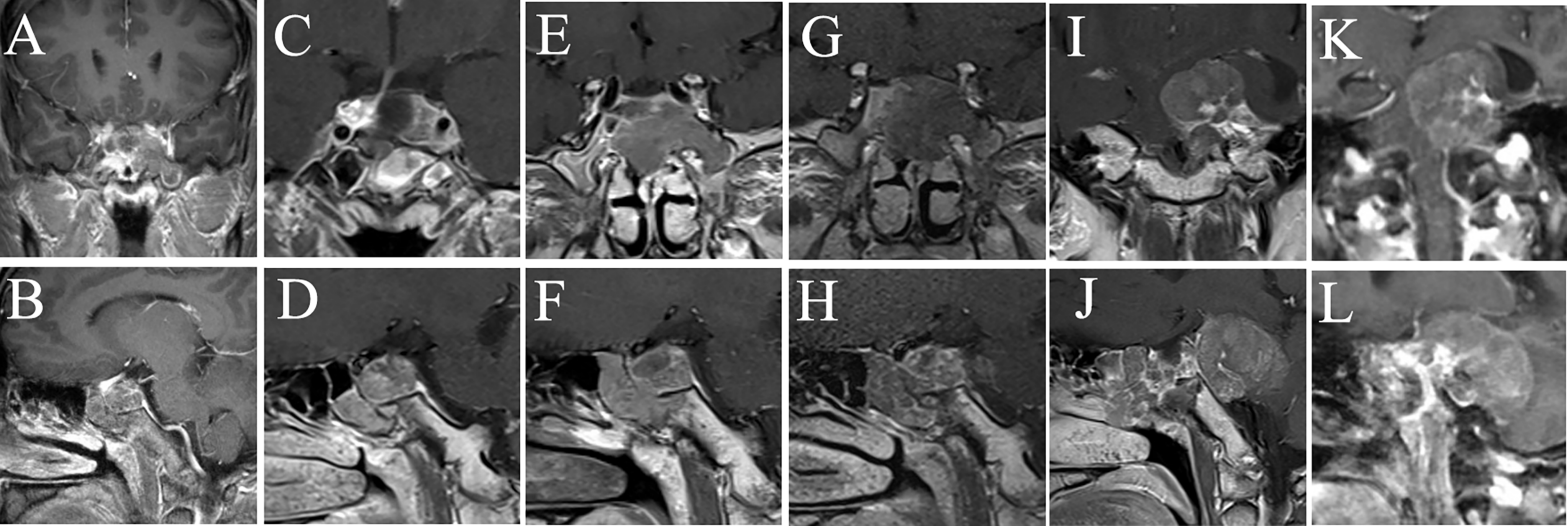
MRI examination of case 1. The images show the patient’s coronal and sagittal MRI of the head before (A, B), one week after (C, D), six months after (E, F), eight months after (G, H), sixteen months after (I, J), and seventeen months after (K, L) the fourth surgery, respectively.
